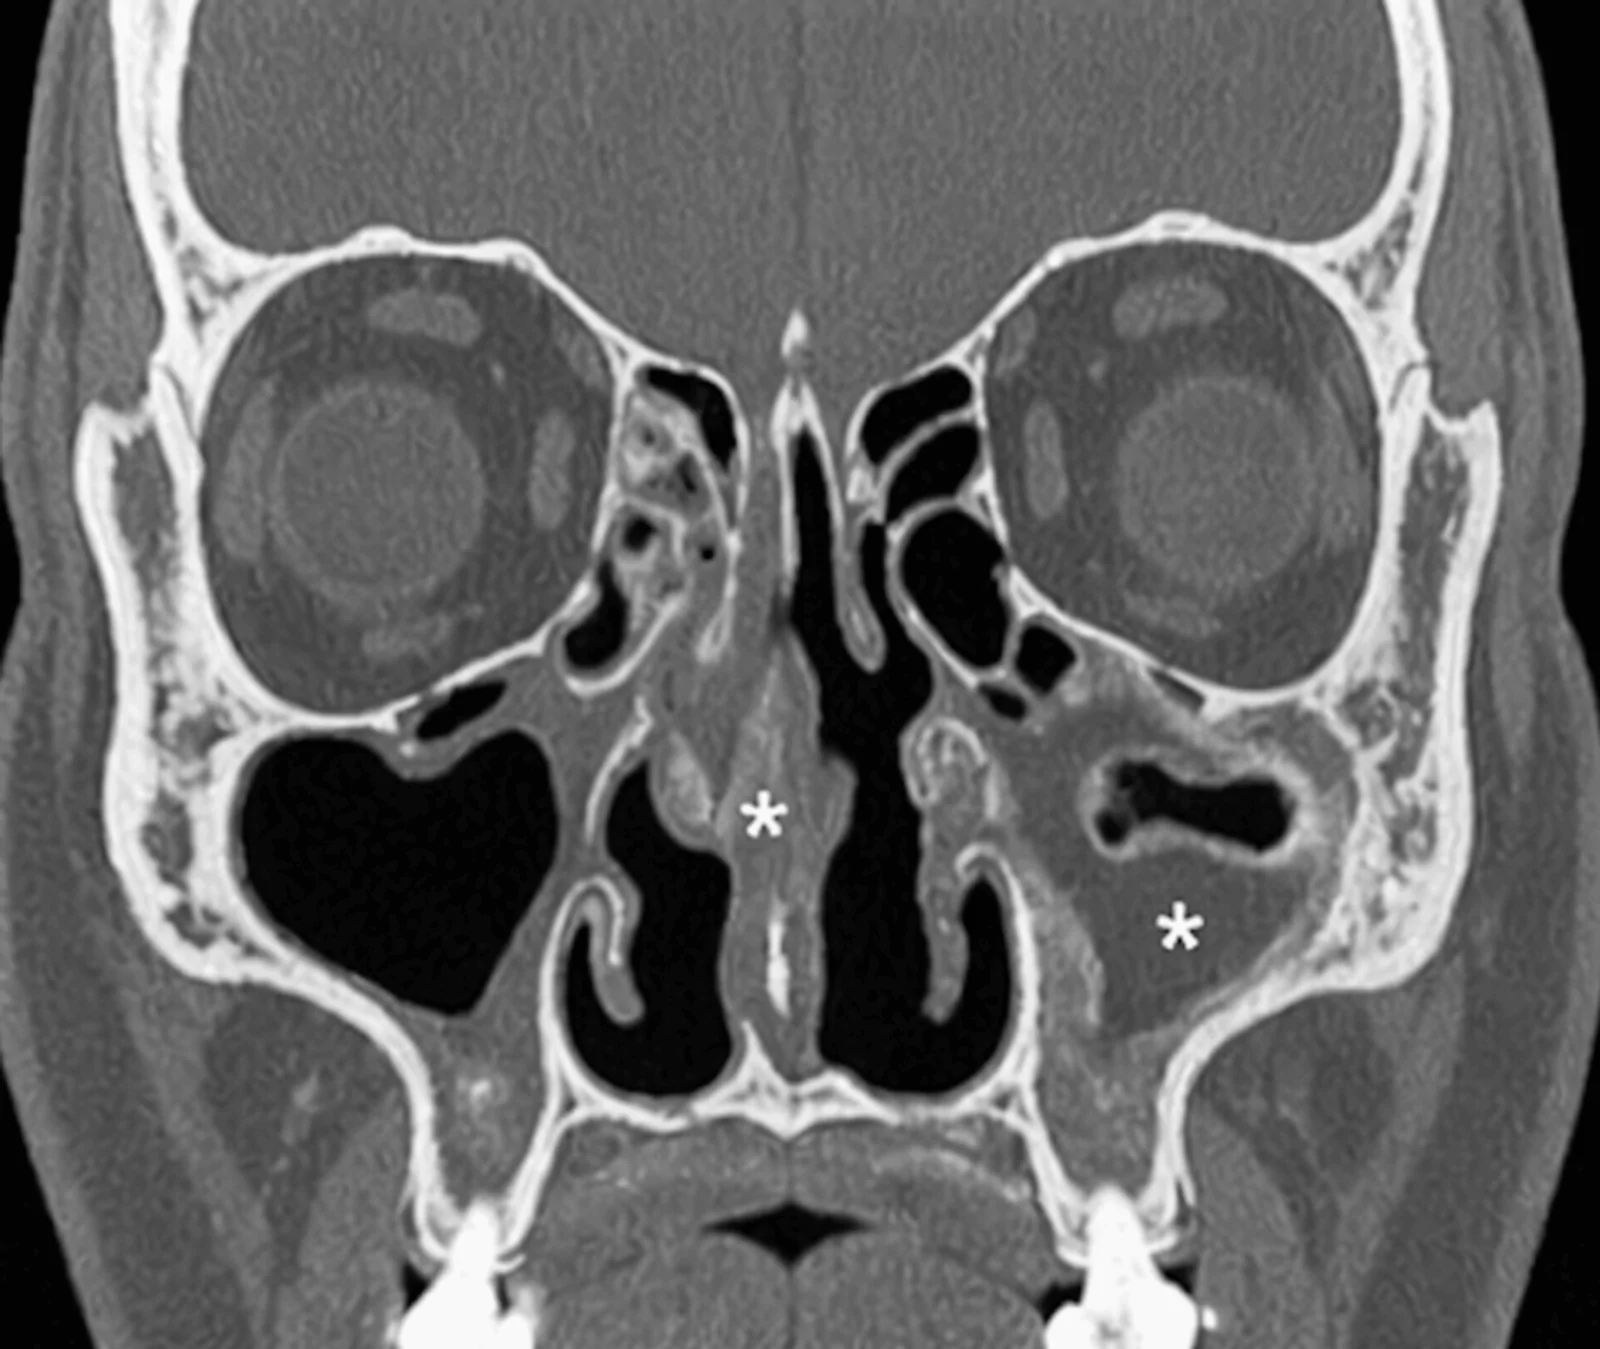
Computed tomography of paranasal sinuses Mucosal thickening and soft tissue in the nasal cavity and paranasal sinuses with bony destruction of the nasal septum (asterisk) and adjacent bony septations
